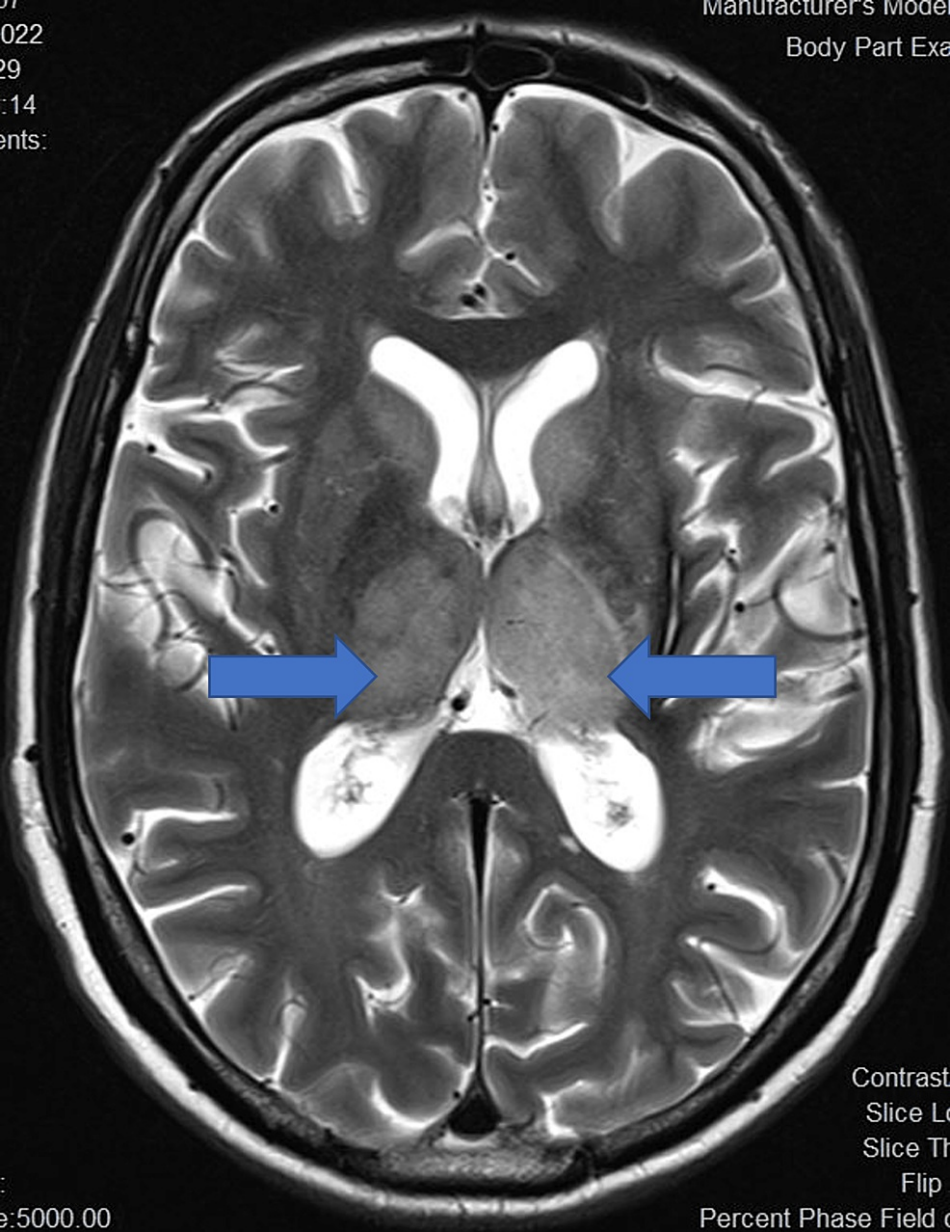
T2 axial MRI image showing bilateral enlarged thalami with hyperintensity MRI: magnetic resonance imaging
